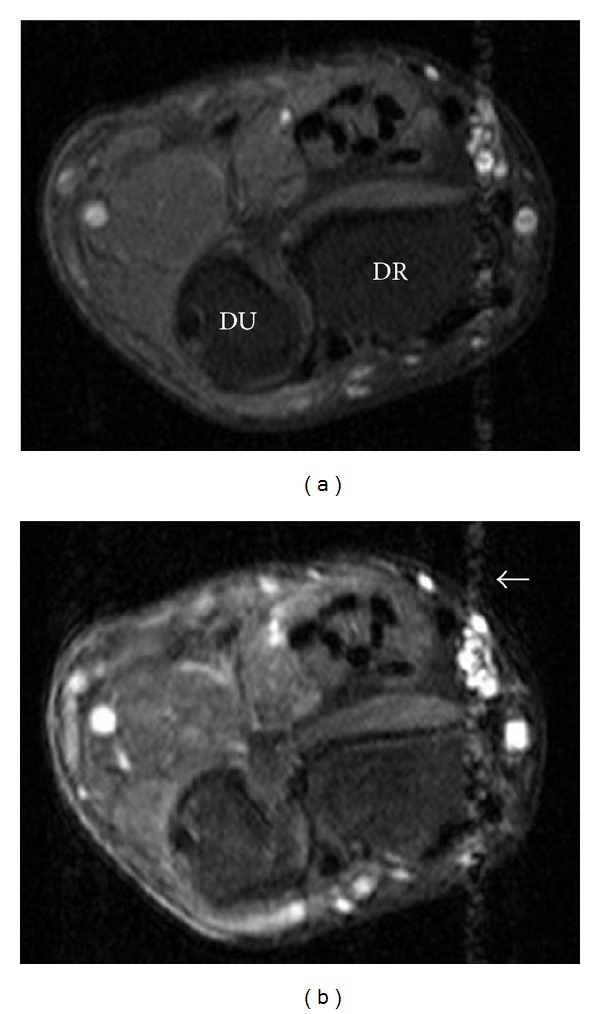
Axial T1-weighted wrist MRI with fat suppression—(5a) precontrast, (5b) postcontrast: the majority of the lesion (seen more clearly on T2-weighted image; Figure 2) showed no significant enhancement. Pulsation artifact from radial artery (white arrow). DR: distal radius; DU: distal ulna.
